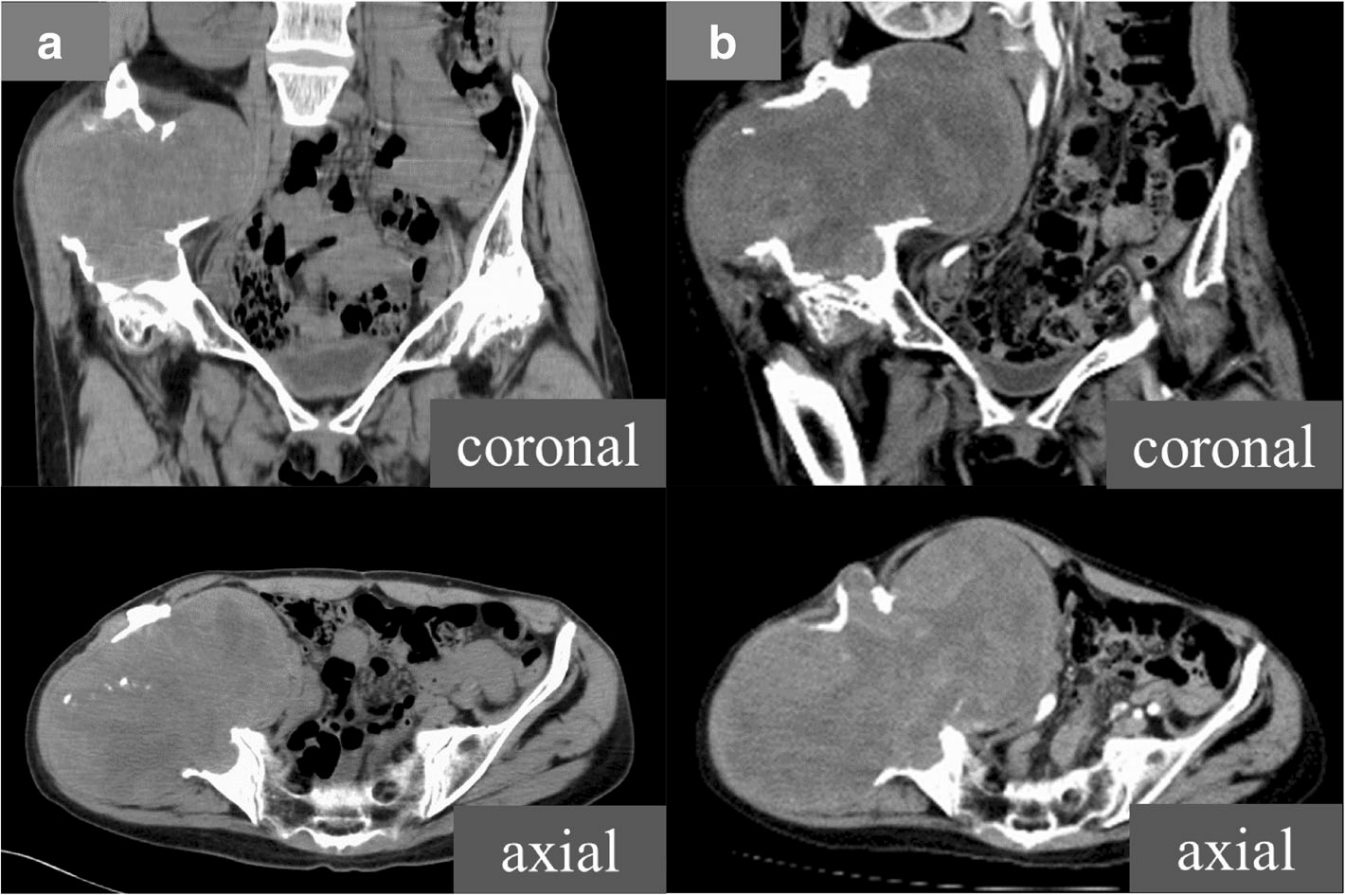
Computed tomography of the ilium at initial presentation (a) and 2.5 years after diagnosis (b). Although a consecutive selective arterial embolization program was performed, the lesion became significantly larger in 2.5 years
